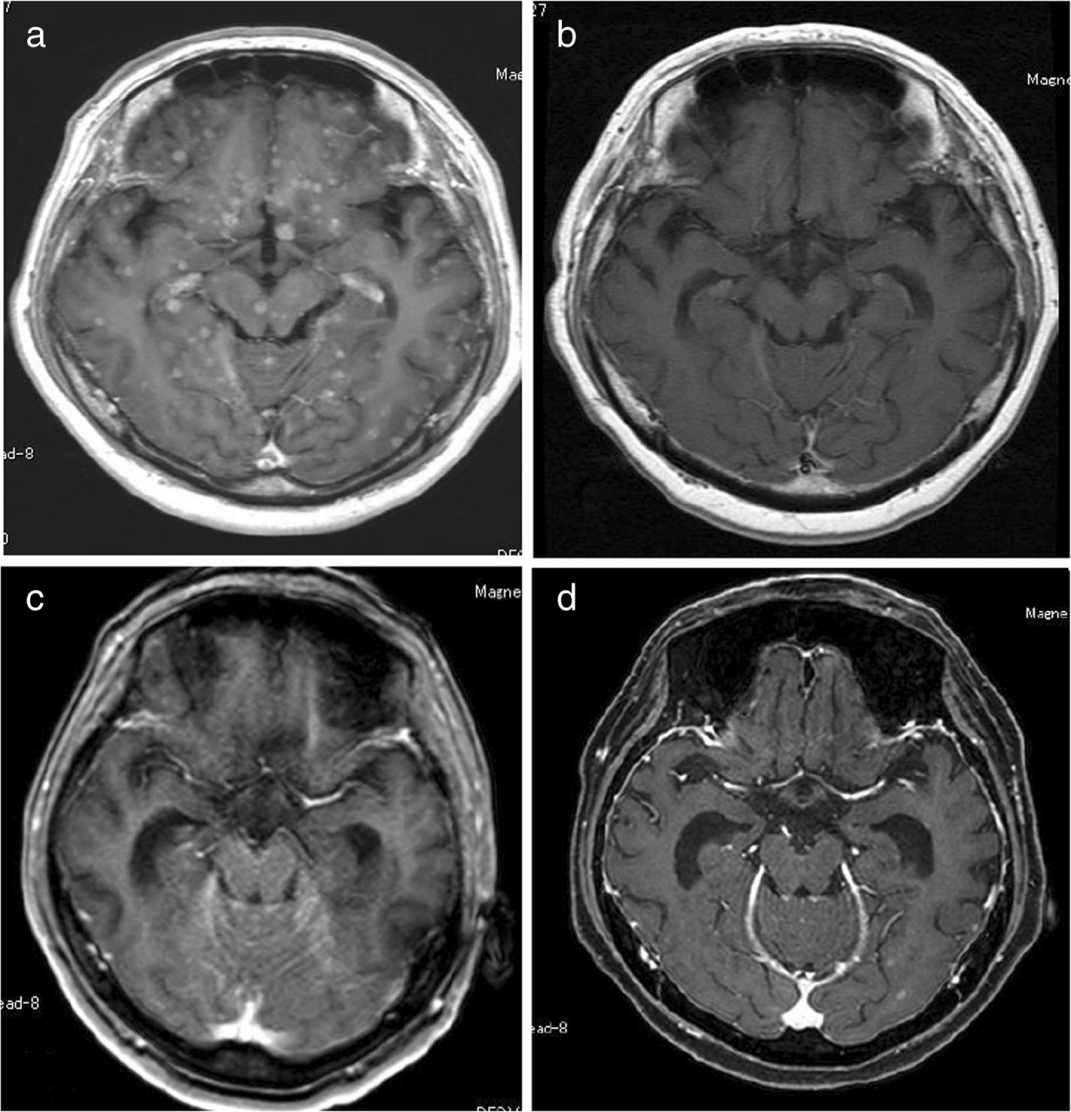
Brain magnetic resonance imaging (T1 gadolinium). a) Multiple, enhanced miliary nodules were observed. b) Following whole-brain radiotherapy and erlotinib treatment, the complete disappearance of the metastatic lesions was observed. c) Following docetaxel, an intensive signal in the cisterna of the posterior cranial fossa indicating carcinomatous meningitis was observed. d) After 1 month of the rechallenge of erlotinib treatment.
